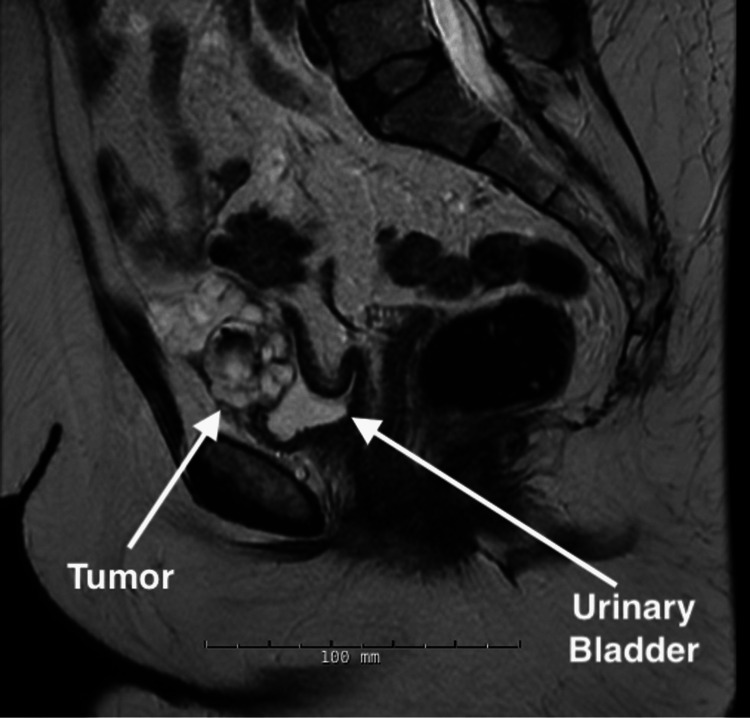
Sagittal MRI depicting the urachal mucinous cystadenocarcinoma in relation to the urinary bladder. MRI: magnetic resonance imaging
